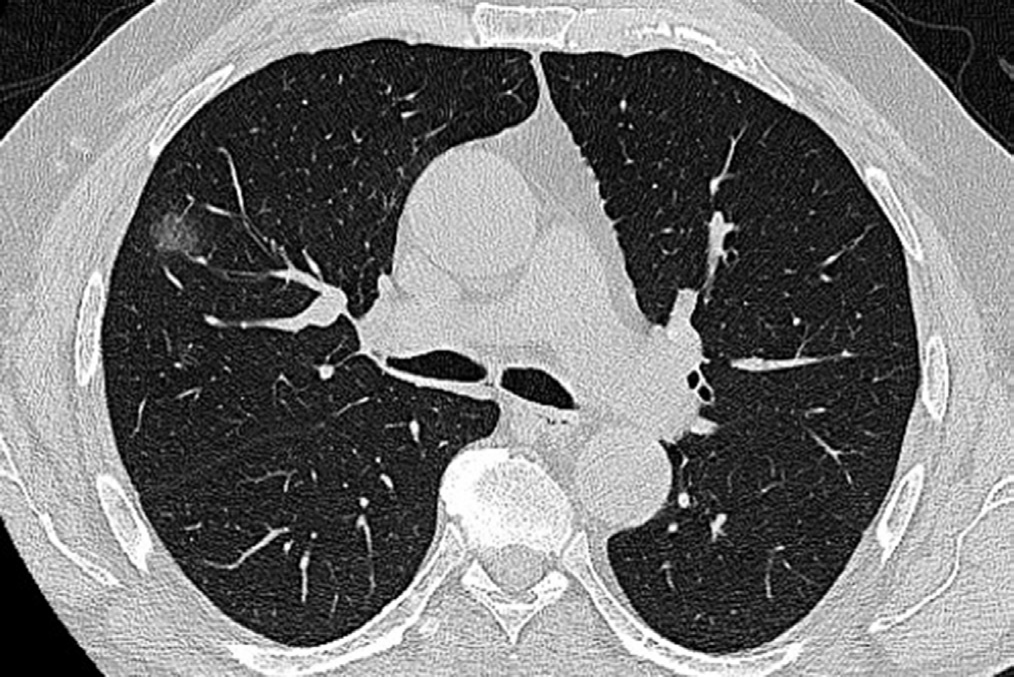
A 54-year-old female COVID −19 patient (2 days since symptoms onset) infected by Omicron variant. CT scan shows single ground glass opacity with rounded appearance, in the right upper lobe.
